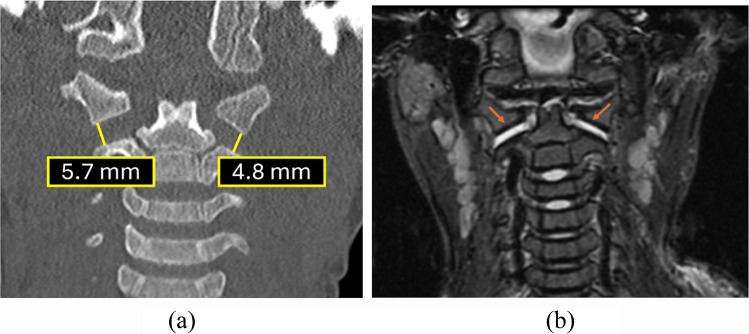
A 3-year-old child involved in a car accident. (a) Coronal CT demonstrates an increased atlanto-axial interval on both sides (normal <3.9 mm). The occipital condyle–C1 interval was also widened (not measured on this image). (b) Subsequent coronal MRI reveals edema within the occipital condyle–C1 joints and in the atlanto-axial interval (*orange arrows*), indicative of ligamentous injury
